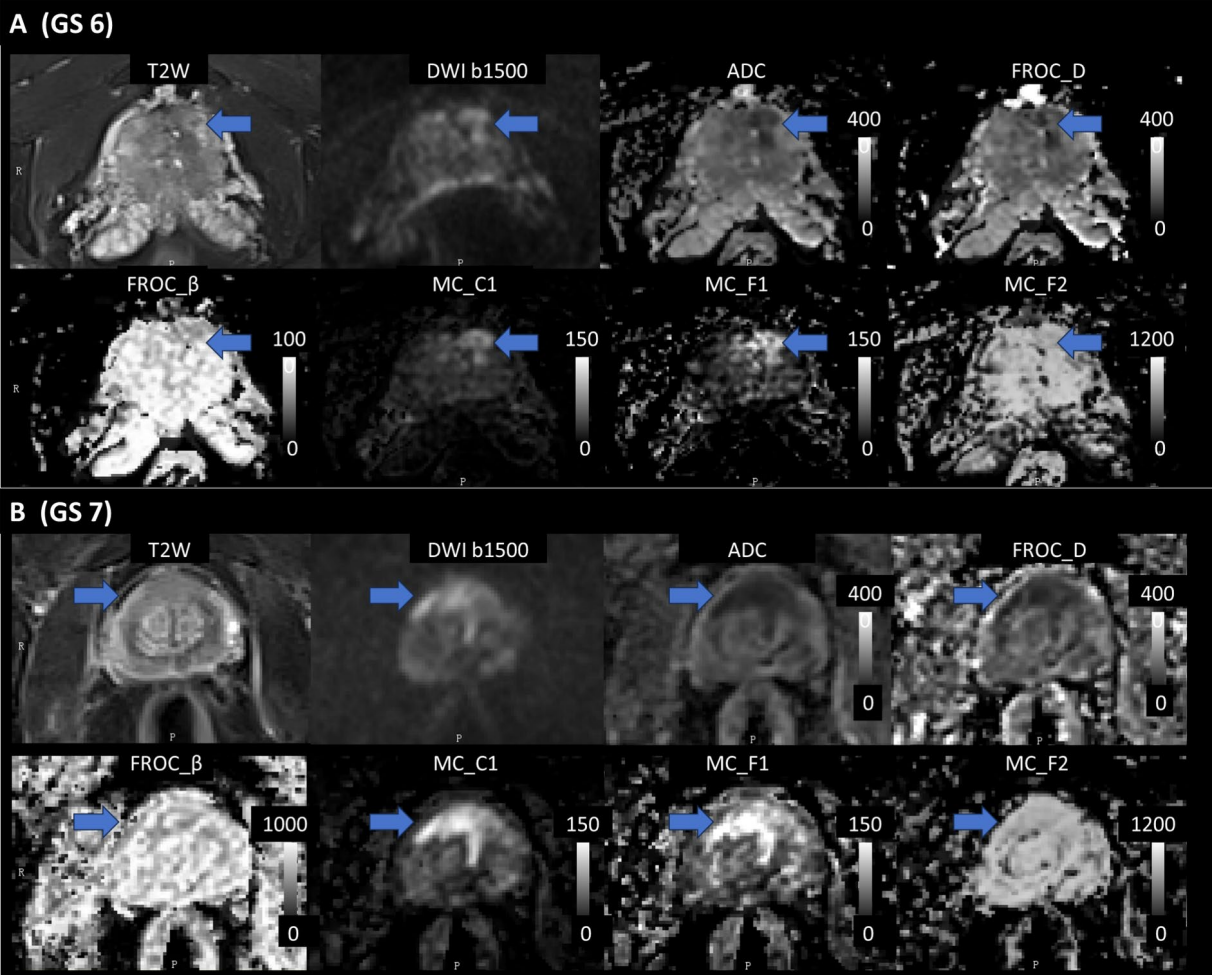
Axial images of two patients with PCa: GS 6 (A) and GS 7 (B). Displayed are the T2-weighted images, DWI images at b 1500, conventional ADC maps, D and β parameters from the FROC model, and C1, F1, F2 parameters from the MC model. The blue arrow indicates the location of prostate cancer (Color figure online)
